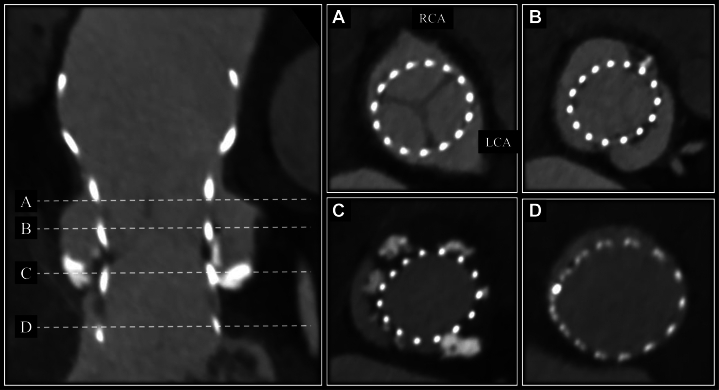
Postoperative Cardiac Computed Tomography Findings (A) Near the sinotubular junction. (B) Upper level of the sinus of Valsalva. (C) Lower level of the sinus of Valsalva. (D) Left ventricular outflow tract.
